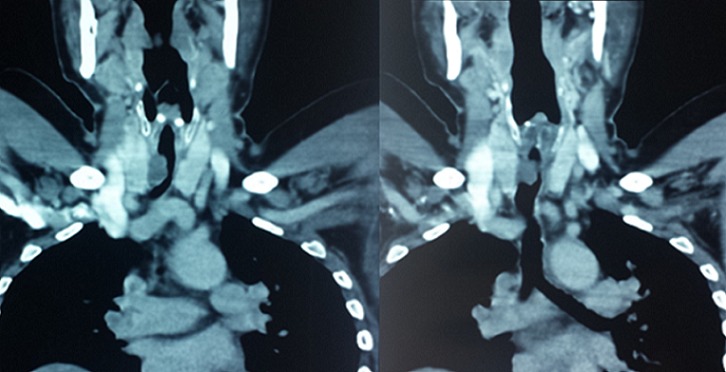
Coronal reformatted CT image showing a large polypoid intra-luminal mass arising in the trachea within 1 cm of the larynx, causing obliteration of 70% of the lumen
